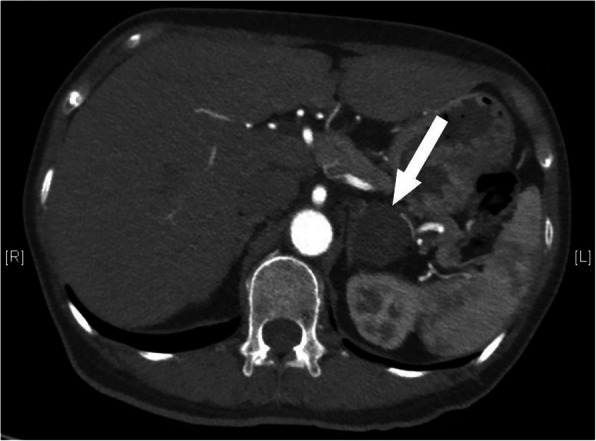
Abdominal CT. Left adrenal mass in 2017 (arrows). CT: computed tomography
